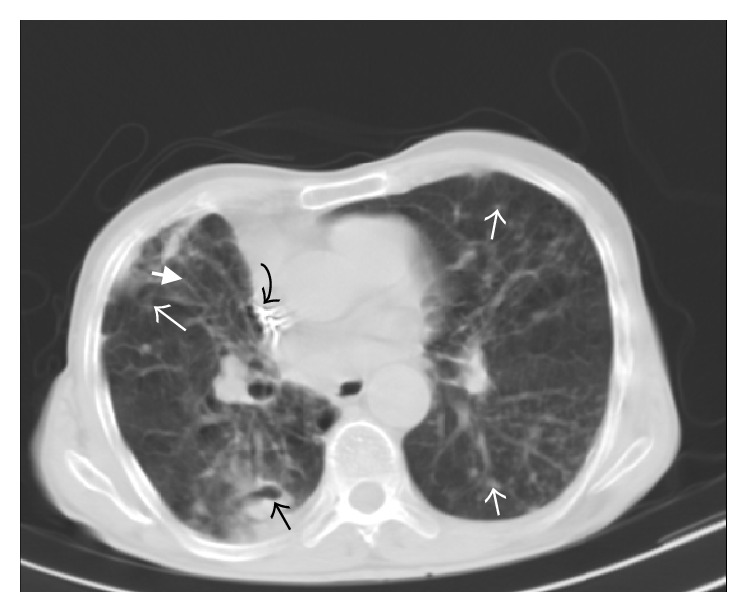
88-year-old male. Spiral CT scan at the level of aortic root (lung window). Cavitary nodule in right lower lobe (black arrow) along with nodular infiltration in left lower lobe, lingula, right lower lobe, and right middle lobe (white arrows). Note also cylindrical bronchiectasis in right middle lobe (thick white arrow). The hyperdense focus in superior vena cava was related to cardiac pacemaker (curved arrow).
